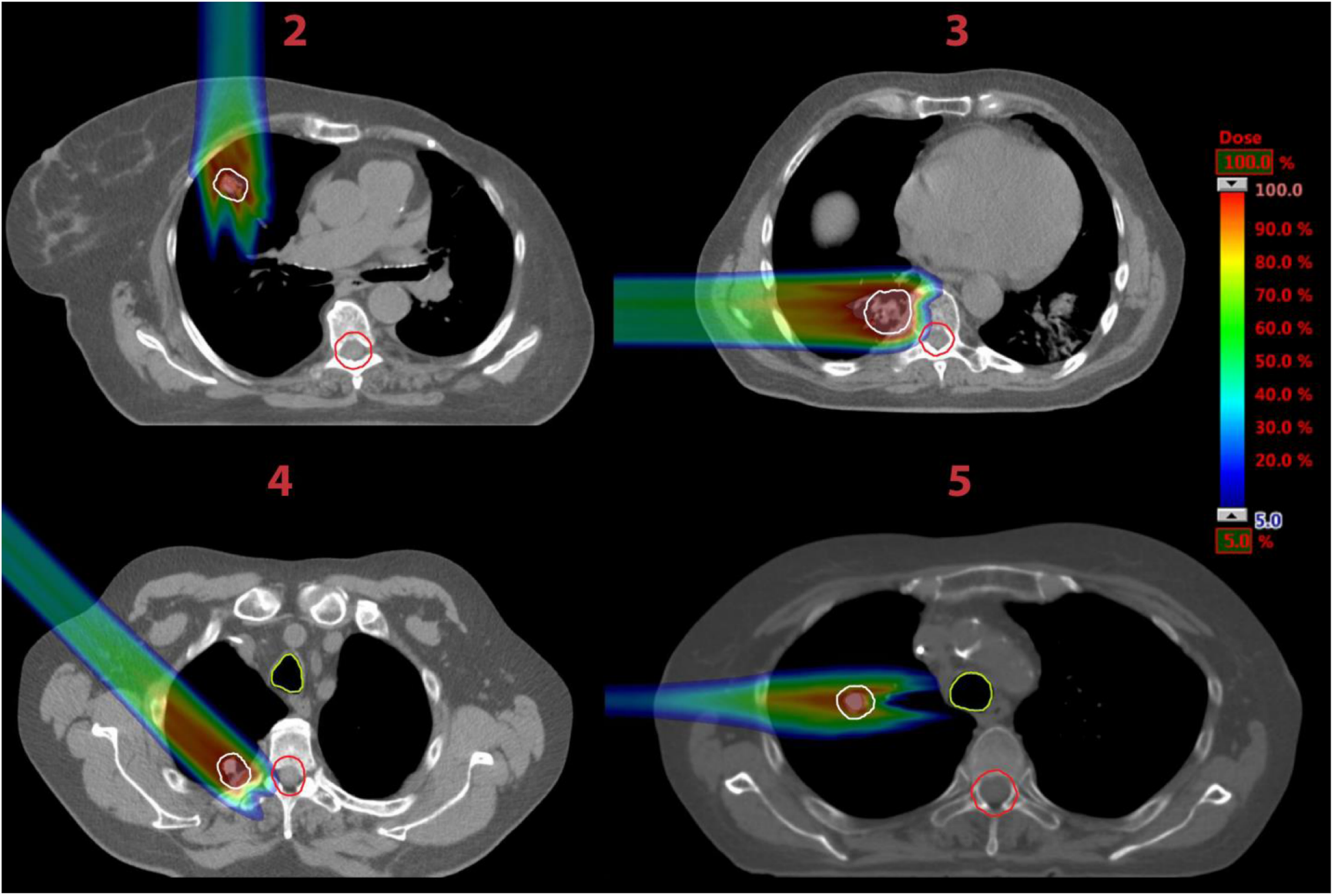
CT slices of the remaining patients. The patient’s numbers are marked in red. For each patient one exemplary plan is shown. The CTV is marked in white, trachea in light green and spinal cord in red. On the right a color bar is given indicating the relative dose
